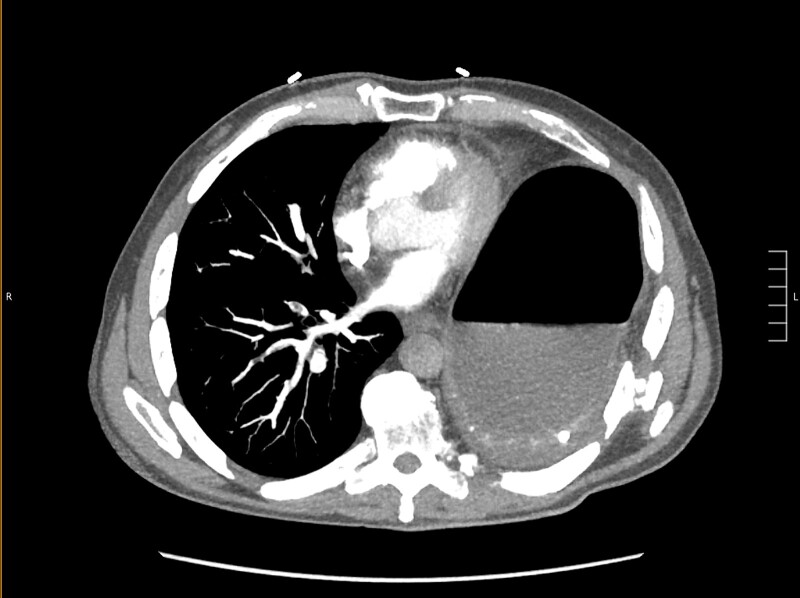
Axial CT showing previously fractured ribs, the “dependent viscera” sign, and thoracic fluid abutting abdominal viscera.
